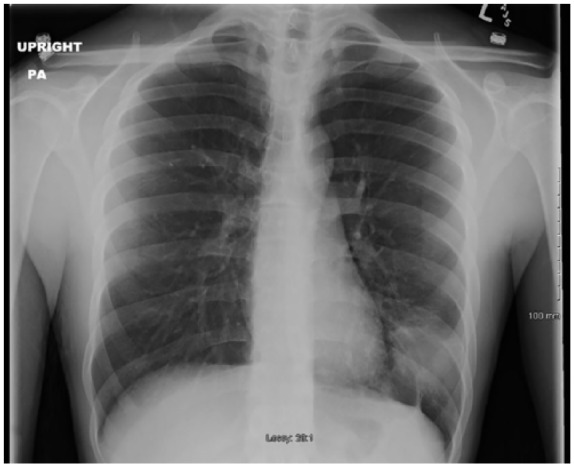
Chest X-ray on admission for case 1.
